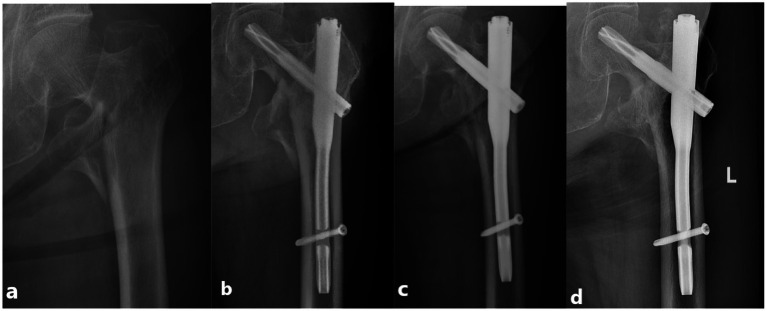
PFNA internal fixation in a 86-year-old male patient with a left intertrochanteric fracture. The patient experienced pain in the left hip and limited movement after the fall, and was unable to walk. At the last follow-up at 14 months after the operation, the Harris score was 85 points and the patient can walk independently. (a) X-ray image showing intertrochanteric fracture of the femur after the injury. (b) Postoperative X-ray showing appropriate fracture reduction. (c) X-ray image showing appropriate internal fixation position evolution 3 months after the operation. (d) X-ray image showing appropriate fracture healing 12 months after the operation.
